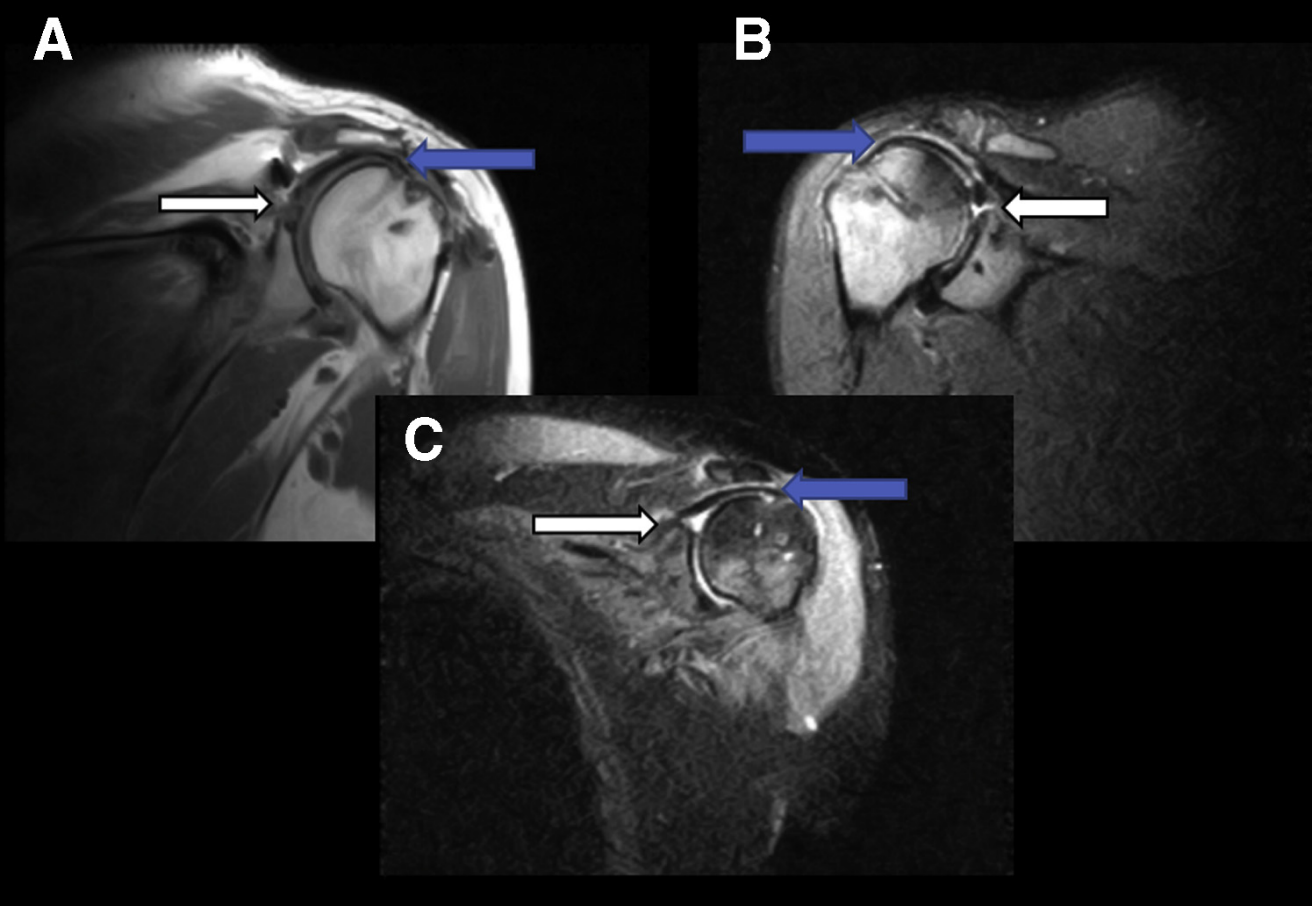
Three completely intact grafts (A, B, and C). These images represent intact grafts on postoperative MRIs after superior capsular reconstruction. The blue arrows denote intact homogeneous graft that can be seen spanning from glenoid to humeral head. White arrows denote heterogenous signal at glenoid, which could represent a normal finding postoperatively or incomplete incorporation at the glenoid graft interface. (A) T1 coronal of left shoulder. (B) T2 inversion recovery coronal of left shoulder. (C) T2 inversion recovery coronal of right shoulder. (MRI, magnetic resonance imaging.)
